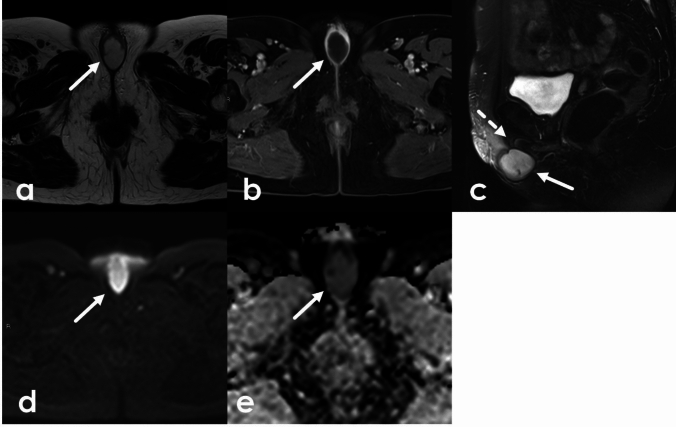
68-Year-old female with clitoral enlargement. Axial (a) and sagittal T2WI (c) demonstrates a T2 hyperintense lesion (white arrow) located inferior and slightly anterior to the glans of the clitoris (dashed white arrow). Post-contrast T1WI demonstrates rim-enhancement (b), and DWI/ADC demonstrates diffusion restriction of this structure (d, e) consistent with clitoral hood abscess
